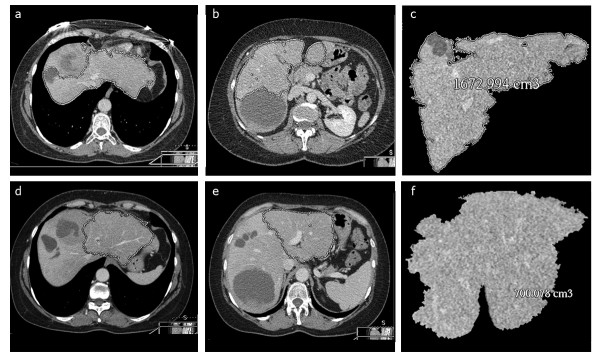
CT volumetry of the liver. (d) through (f) The volume of segments II, III, and IV_B_, which were going to be spared during surgery, were calculated and (a) through (c) divided by the volume of the functional liver before surgery. (a) and (b) The lesions were excluded for the calculation of the functional liver volume.
